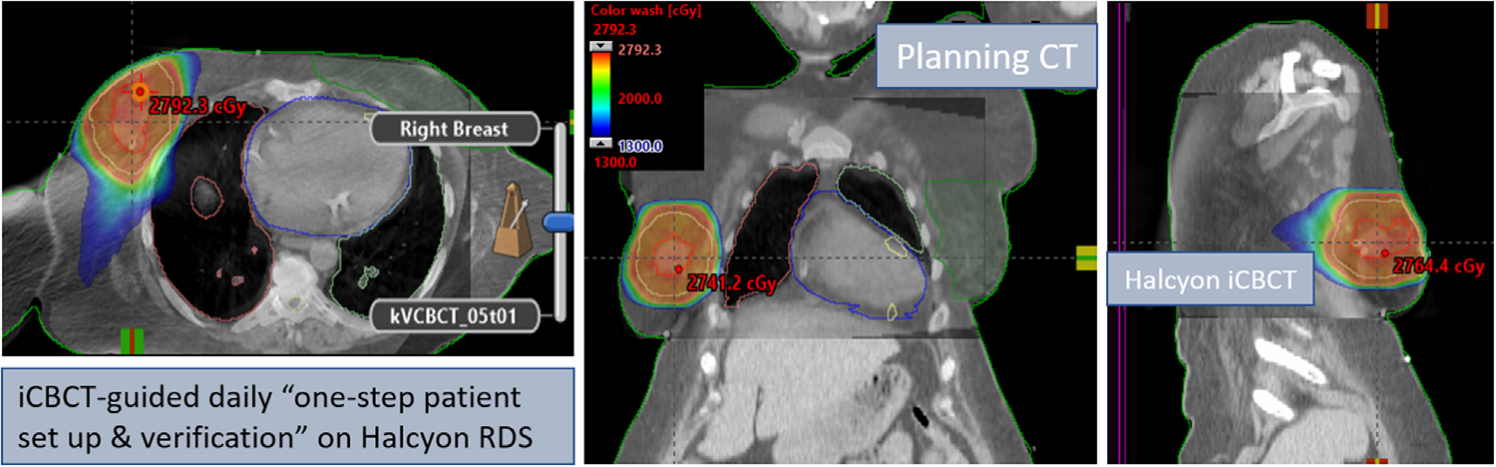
Axial, coronal, and sagittal views of Halcyon kV‐iCBCT images (see inset) co‐registered with planning CT images (see back of coronal and sagittal views) used for image‐guided APBI treatment on Halcyon. In addition to anatomical landmarks, the planned dose cloud was superimposed for this treatment. Halcyon kV‐iCBCT images were acquired in the treatment position on breast board. The 3D soft‐tissue matching was performed via auto‐registration of online kV‐iCBCT scan with the planning CT followed by manually fine‐tuning for more accurate patient setup corrections.
